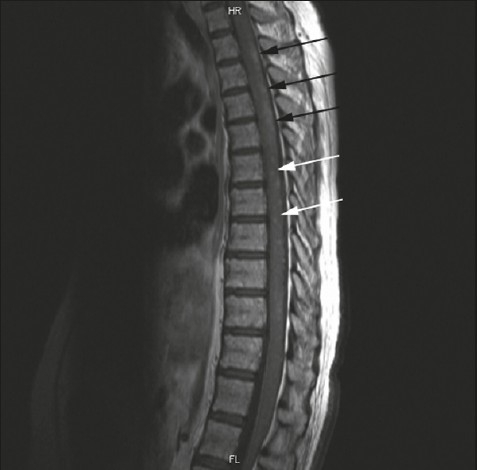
MRI of the thoracic spine (sagittal view T1-weighted post-gadolinium image) showing some multifocal areas of enhancement within the cord that predominantly involve the posterior aspect of the cord (black arrows).There are also extensive focal small enhancing lesions (white arrows).
